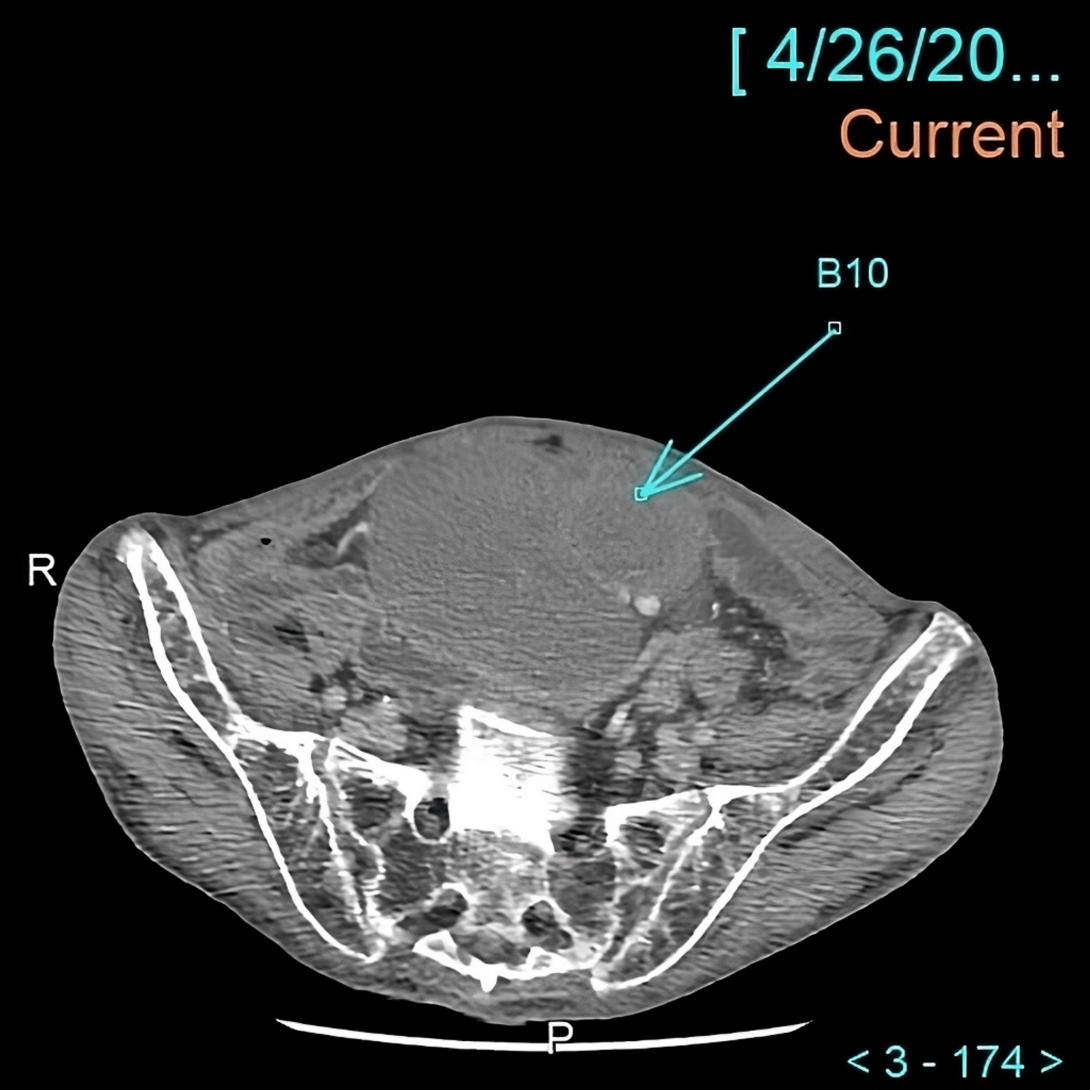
CT abdomen and pelvis done in April 2023 after injection of 80 cc of contrast shows an intraperitoneal lobulated space occupying lesion measuring approximately 11 cm x 7.7 cm with a mean CT number of 37 units (blue arrow).
